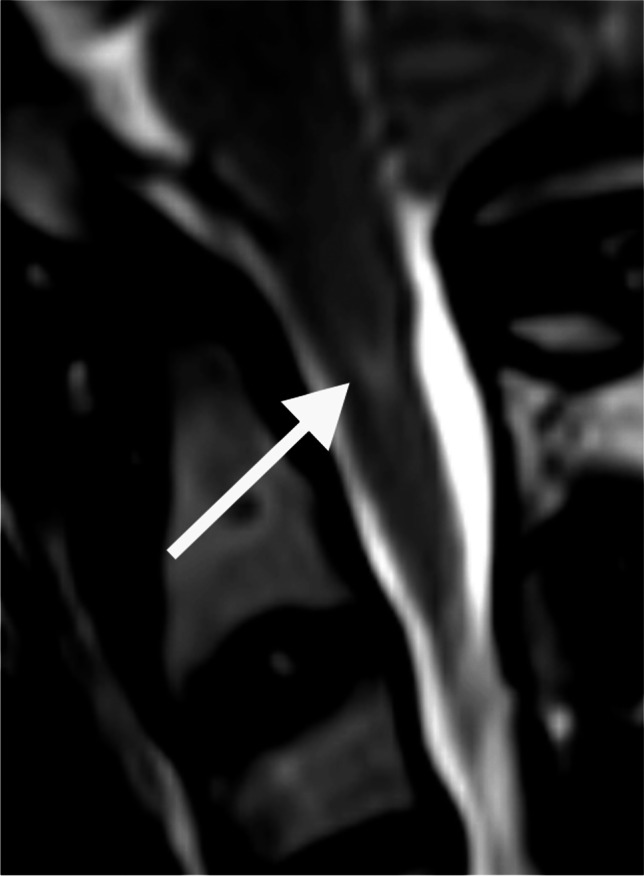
Sagittal T2 magnetic resonance images of the craniocervical junction in a 12-year-old girl with achondroplasia. There is subtle increased T2 signal (*arrow*) without evidence of foramen magnum stenosis
